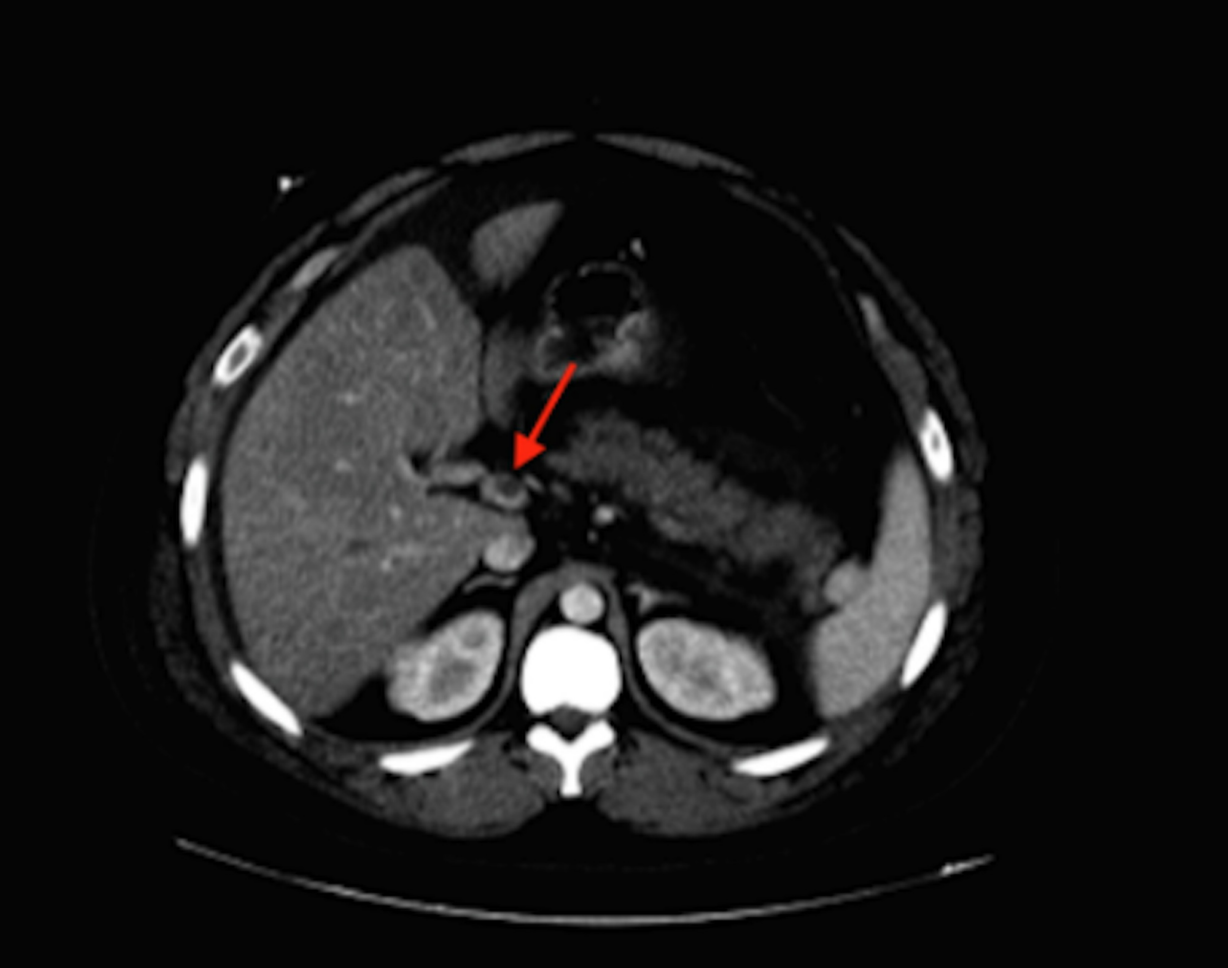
Thrombosis of the hepatic and portal vein in CT abdomen without contrast
